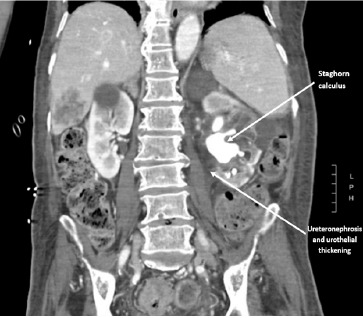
Computed tomography coronal view of a large left staghorn calculus with significant hydroureteronephrosis and urothelial thickening.
